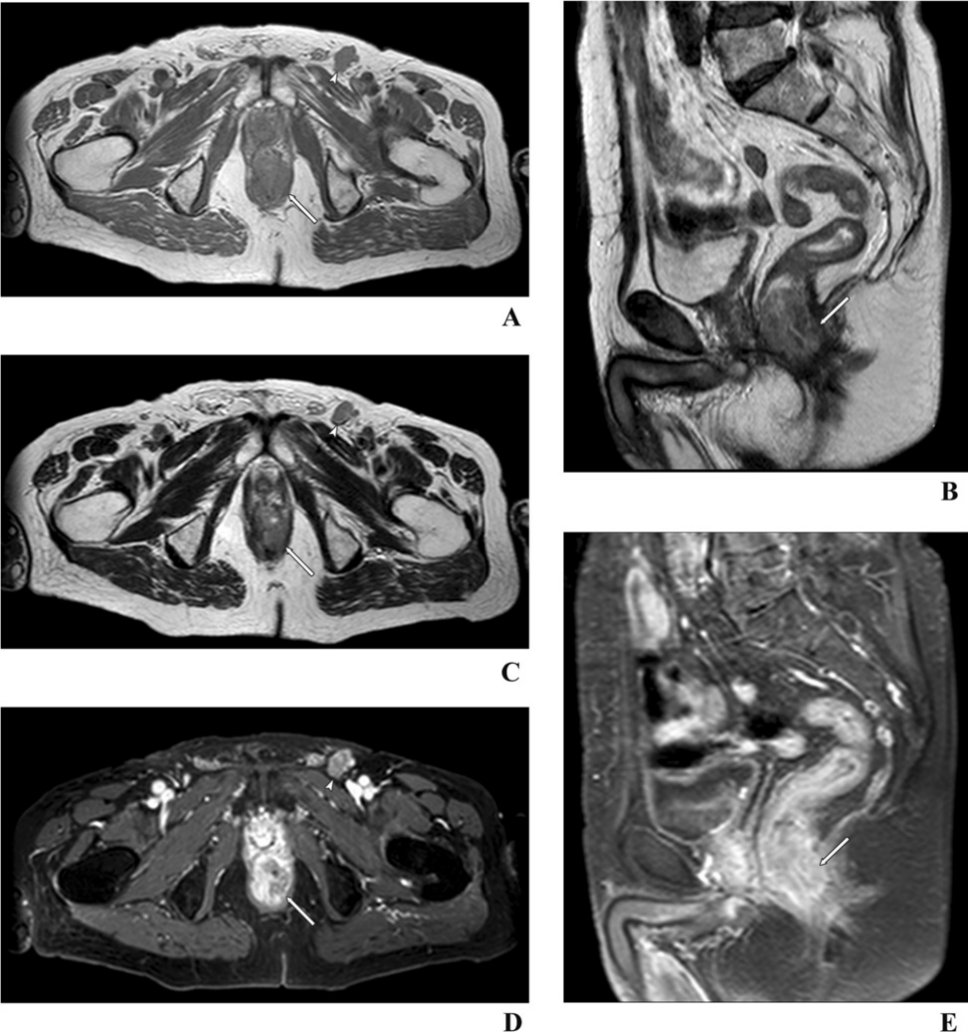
Anal cancer with invasion of the intersphincteric space, external anal sphincter (arrow) and metastasis in the inguinal lymph node (arrowhead). Examination with a 1.5-T pelvic phased array coil. Axial T1- (A), T2- (C) and T1-weighted with fat suppression post-gadolinium-enhanced images (D). Sagittal T2- (B) and T1-weighted images with fat suppression post-gadolinium-enhanced images (E).
